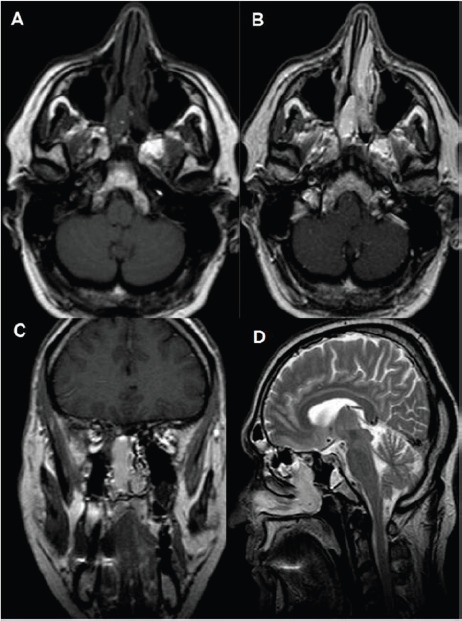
MRI (a) T1WI showing mainly hypointense mass in the right nasal cavity and posterior ethmoid air cells with retained secretions and inflammatory changes to the left ethmoid air cells (b) T1WI GD axial and (c) Coronal images showing the expansive soft tissue mass well-defined with intense enhancement (d) T2WI sagittal plane showing high signal intensity of the lesion.
